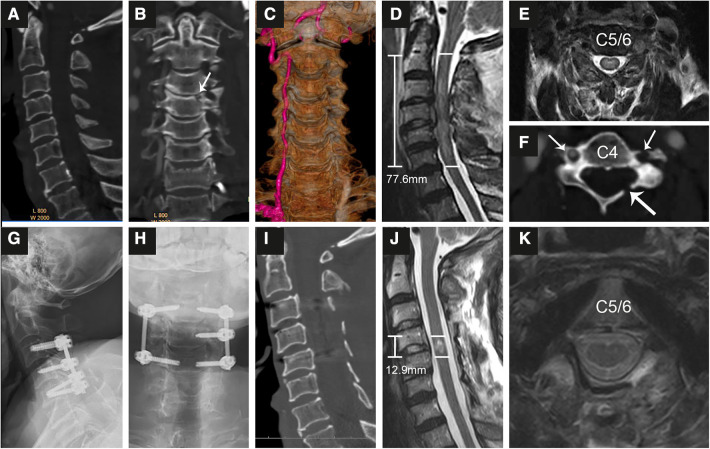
Representational preoperative (A–F) and postoperative (G–K) images of a 68-year-old male patient who sustained an A1 type fracture and spinal cord injury. His AMS was 11 and AIS grade B. Midsagittal (A), coronal (B,C) and axial (F) enhanced CT indicated an A1 type fracture at C4 (B) accompanied by fractures of the left lamina (F, long arrow) and transverse foramen (short arrow) and injury to the left vertebral artery (C). MRI (D,E) showed SCI with an intramedullary lesion length (IMLL) of 77.6 mm. The subarachnoid space (SAS) was absent from C3 to C5 (D). Laminoplasty of C3–7 and pedicle screw fixation from C3–C5 were performed in this patient 9 h after trauma. Postoperative x-rays (G,H) and CT (I) showed good screw positioning and significant enlargement of the osseous spinal canal. Postoperative MRI indicated an IMLL of 12.9 mm (J), successful decompression (J), and reduced edema 12 days after trauma. One year after injury, the patient paralysis recovery with an AMS of 72 and AIS grade D.
